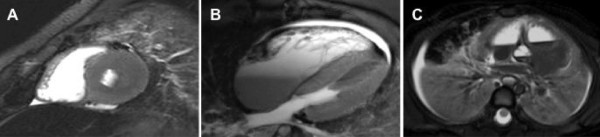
Post-mortem MRI scans for patients with false positive imaging and unexplained autopsy diagnosis. (A) Patient 7 with incorrect diagnosis of hypertrophic cardiomyopathy. Short axis STIR showing asymmetrically increased wall thickness in the posterior left ventricular wall. (B) Patient 9 with incorrect diagnosis of arrhythmogenic right ventricular cardiomyopathy. 4-chamber STIR showing relatively larger RV size in comparison to LV. (C) Patient 6 with incorrect diagnosis of pneumonia. STIR image through lung and heart showing appearance of lung consolidation with associated collapse bilaterally.
